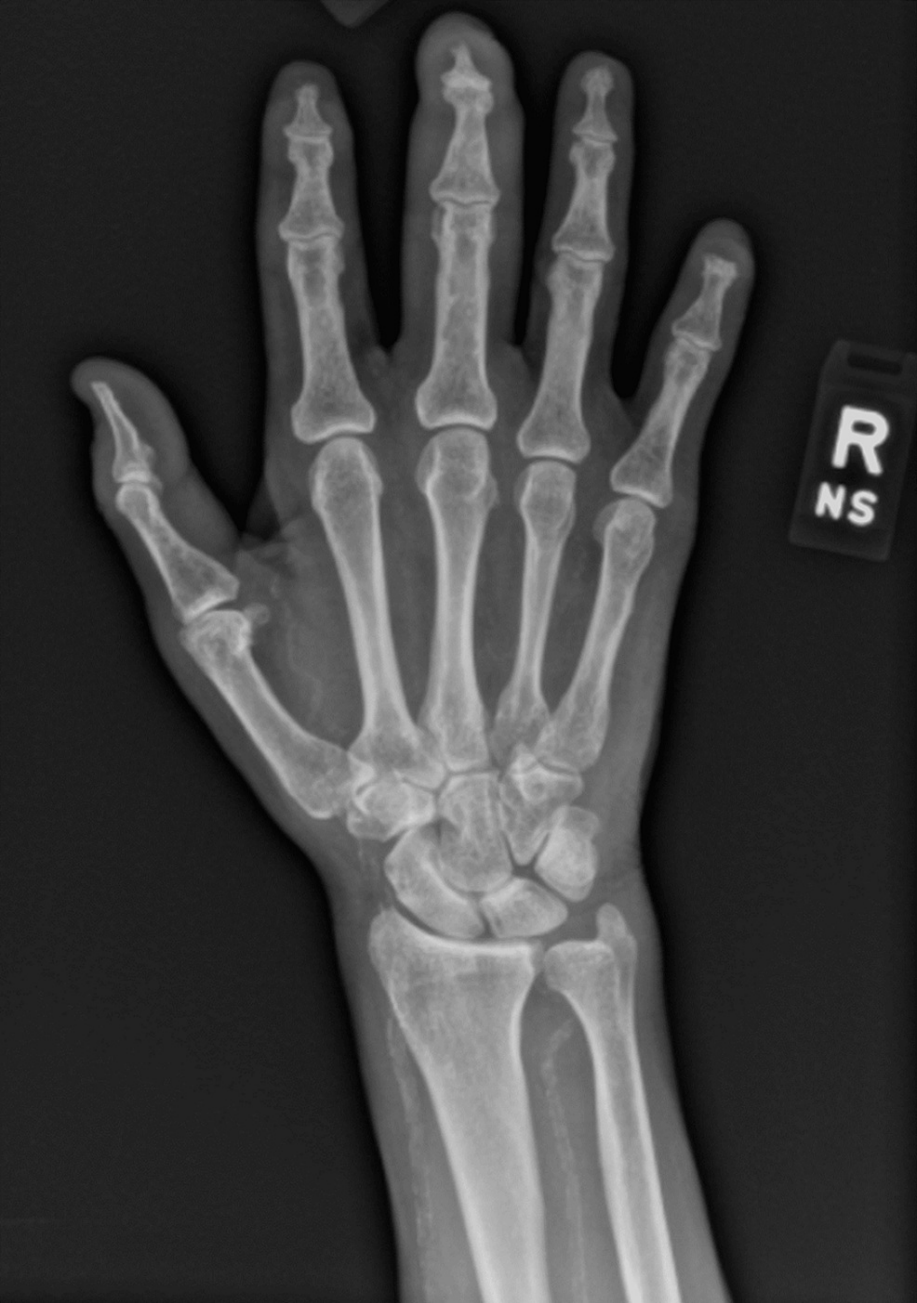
X-ray of right hand. There is absence of the tip of the third digit distal phalanx with a questionable osteomyelitis.
